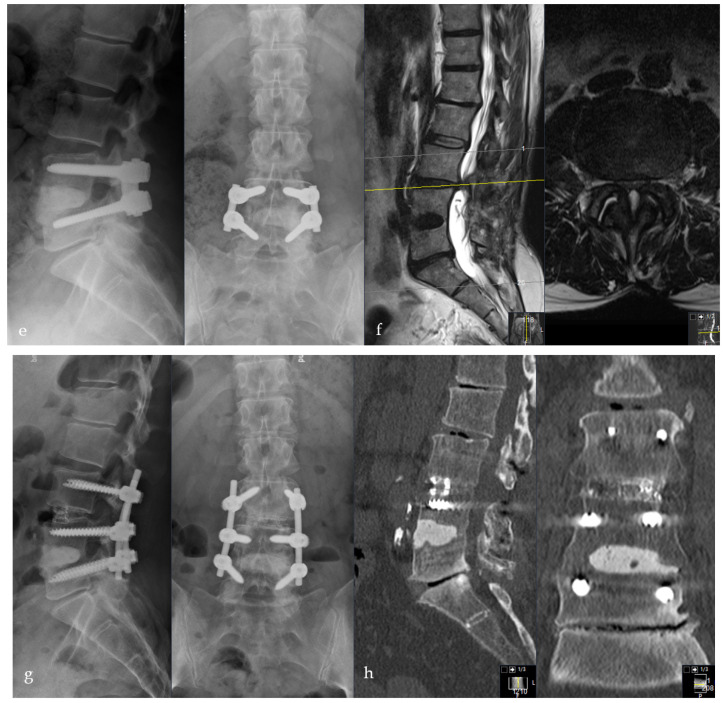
Female patient, 46-years old, pyogenic erosive spondylodiscitis L4/5 with staphylococcus aureus identified as the causative pathogen. Preoperative standing a.p. and lateral X-ray (a), MRI of the lumbar spine (b), and CT scan (c). Postoperative standing a.p. and lateral X-ray after Cement-PLIF L4/5 and postero-lateral fusion (d). Six-year follow-up with standing X-rays a.p. and lateral of the lumbar spine (e). Cranial adjacent segment disease (L3/4) is demonstrated by MRI (f). Adjacent segment fixation L3/4 after preoperative exclusion of reinfection by open biopsy with postoperative standing X-rays of the lumbar spine six years post-operatively (g). Sixteen-year follow-up CT scan of the lumbar spine with intact Cement-PLIF, anterior and posterior fusion, and infection elimination (h).
